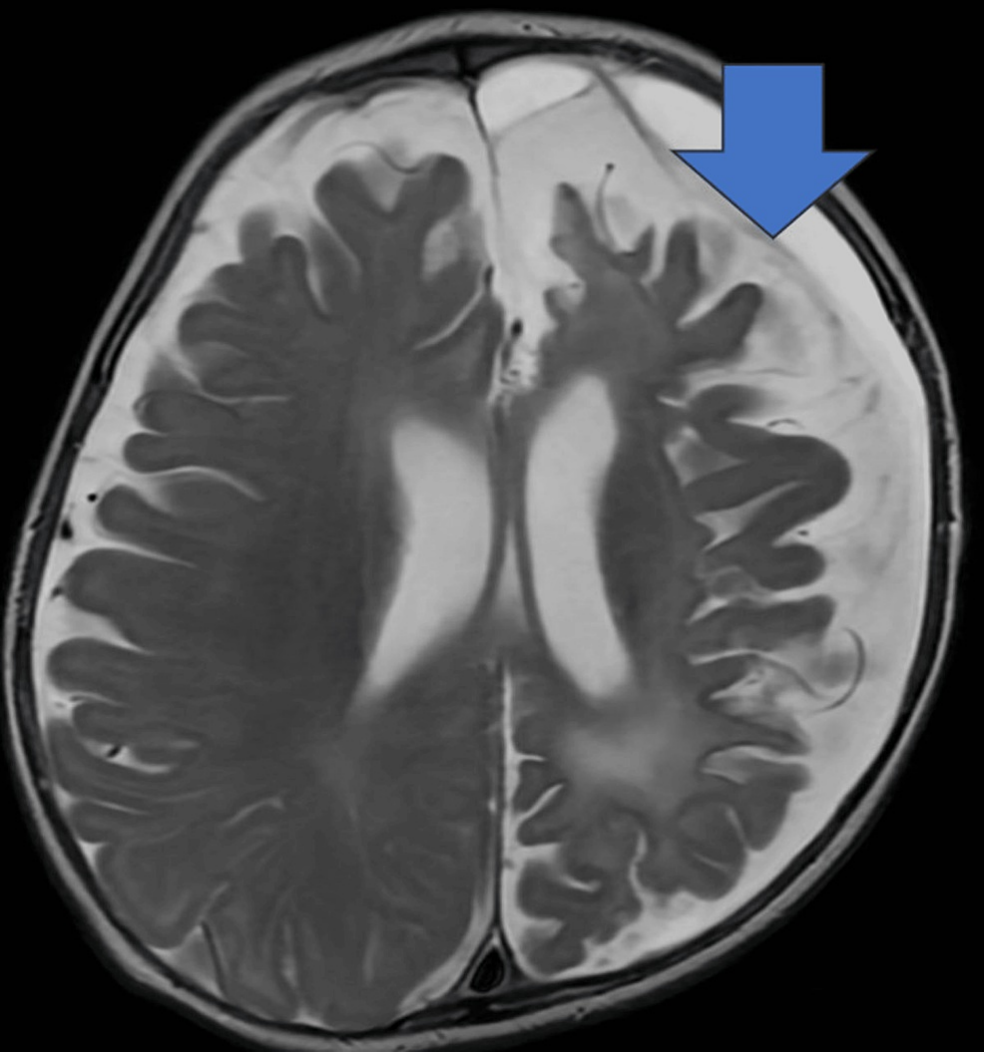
Axial T2 image on follow-up MRI of the brain several months later demonstrates encephalomalacia, gliosis, and volume loss in the left cerebral hemisphere with the development of a likely compensatory subdural hygroma along the left cerebral convexity (Monro-Kellie doctrine). The blue arrow indicated encephalomalacia, gliosis, and volume loss in the left cerebral hemisphere with the development of a likely compensatory subdural hygroma along the left cerebral convexity.
